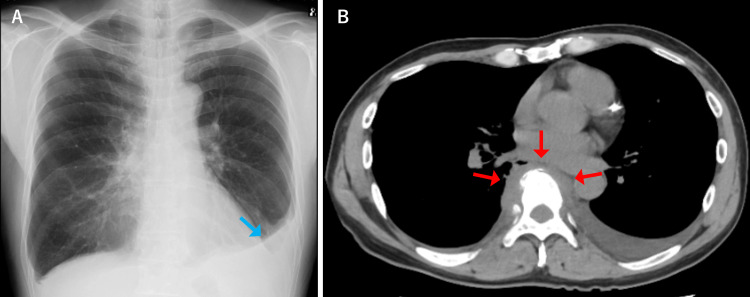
Chest radiography and plain chest CT images on the 22nd day after admission CT: computed tomography (A) Chest radiography shows blunting of the left costophrenic angle, suggesting the appearance of a left pleural effusion (blue arrow). (B) Chest CT shows extension of the soft tissue density around the vertebral bodies to the left side (red arrows)
